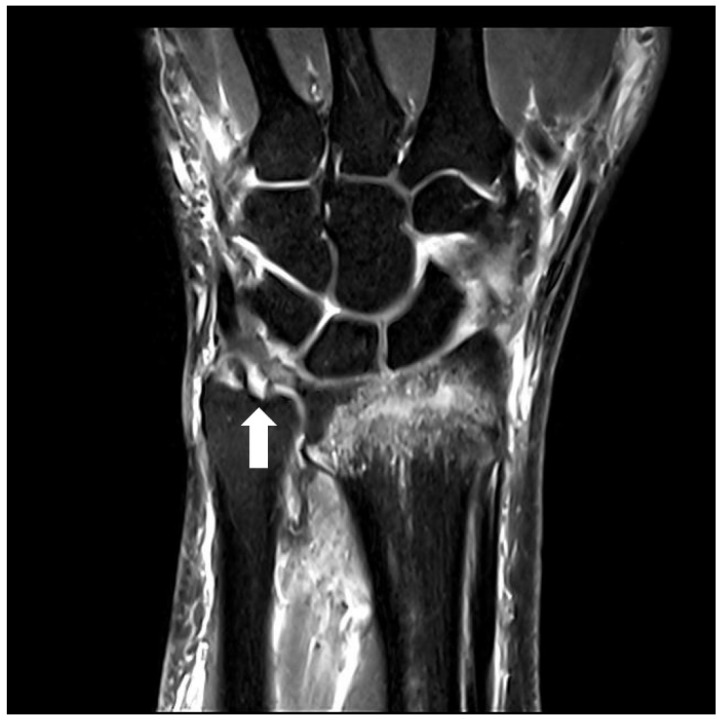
Preoperative magnetic resonance images of the left wrist. Proton density-weighted turbo spin-echo imaging in the coronal plane. The proximal component of the triangular fibrocartilage complex (TFCC) is torn at the foveal attachment (white arrow), compatible with a TFCC foveal tear.
